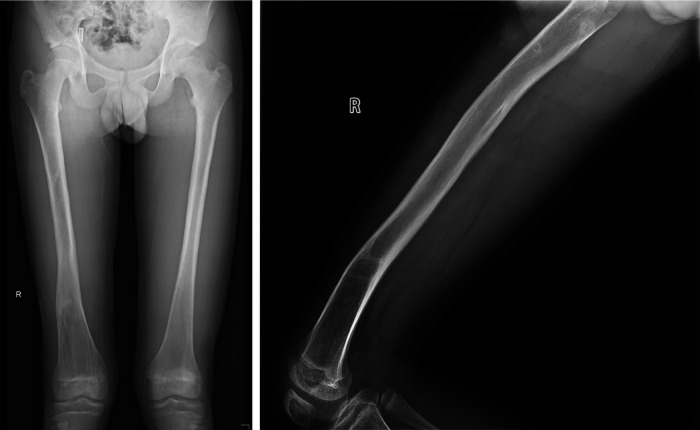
Taken during a 5-year follow-up examination, shows a right lateral view x-ray of the femur, demonstrating equal length of both femurs, good remodeling of the right femur, and slight bending seen on the lateral view.
